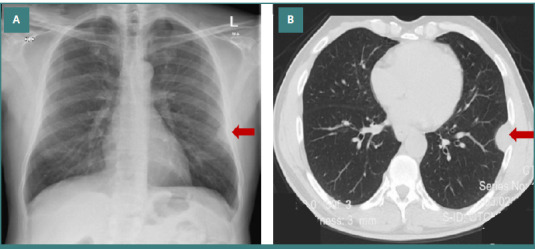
Radiological image of pleural mass. A, Plain chest X-ray showing a left pleural-based mass. B, CT scan highlighting the pleural-based elliptical mass
